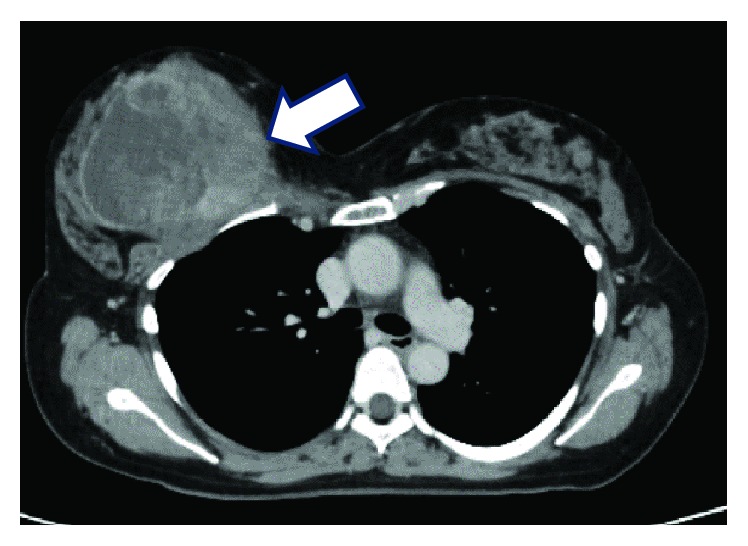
Computed tomography scan showing a 9-cm mass in the right breast (arrow).
